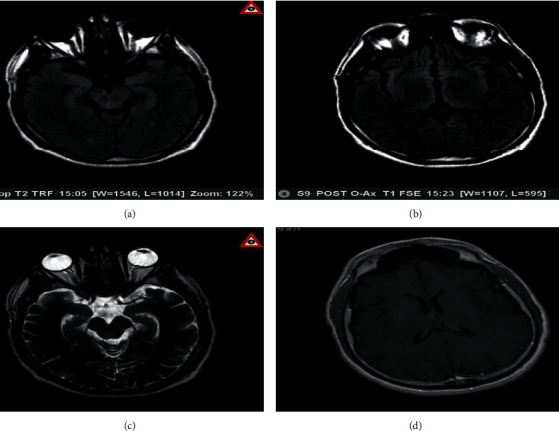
Axial magnetic resonance images (MRIs) one and a half weeks after treatment with immunoglobulin and methylprednisolone demonstrating gyriform swelling of the temporal lobes (a), especially medially, best seen on flair sequences (a, c, d). There is a faint bright signal intensity observed in the bilateral thalami and basal ganglia (c). Vague cortical brightness is also seen. There is no evidence of abnormal contrast enhancement (b). Compared to the earlier study, the degree of bright signal intensity is decreased, though swelling remains. These findings, with cortical, thalamic, and basal ganglia involvement, are typically seen in encephalitis.
